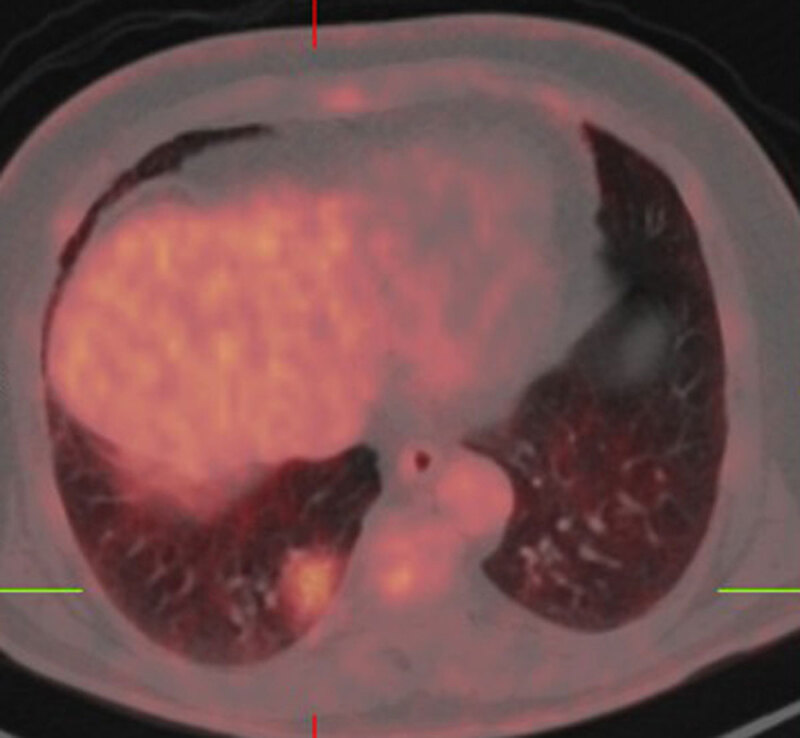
Fused ^18^FDG-PET/CT image in axial plane shows moderate FDG uptake in the subsolid nodule in the right lower lobe.
